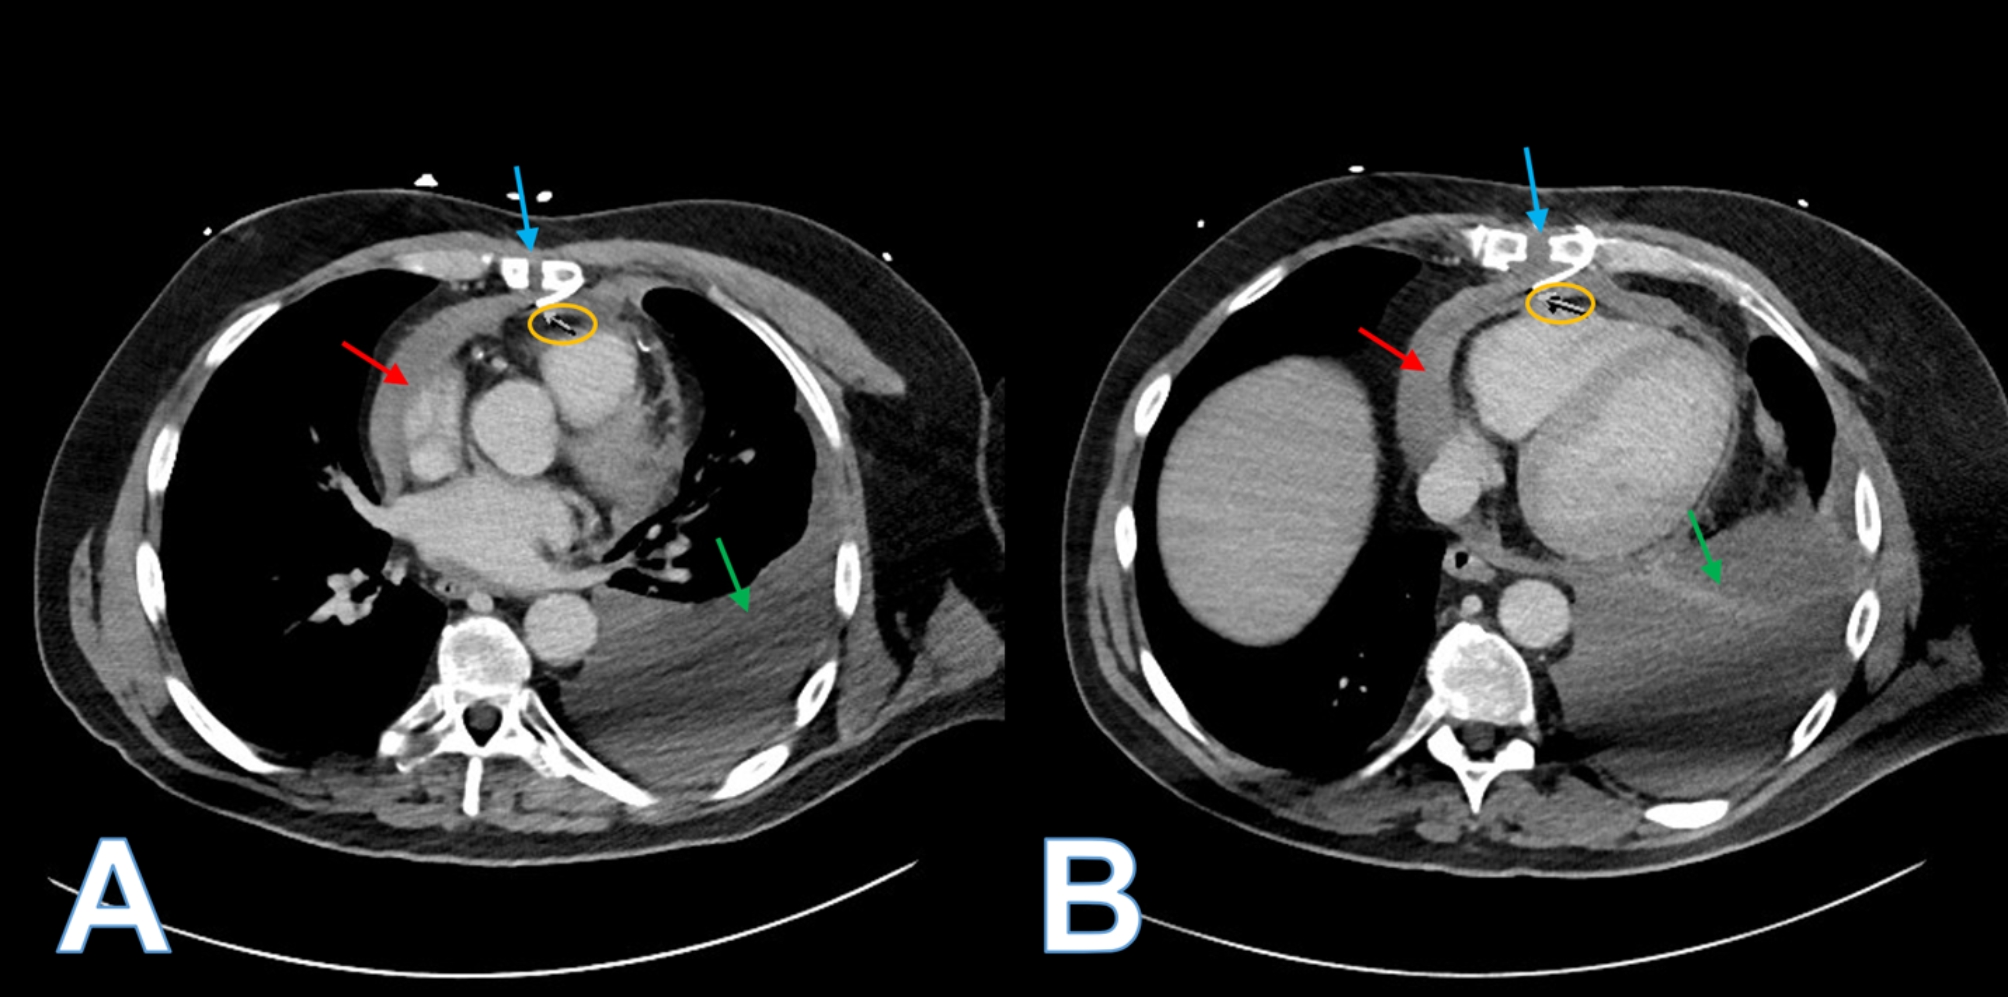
A) Computed tomography angiography at the level of the left atrium demonstrating sternal bone diastasis (blue arrow), fractured sternal wire angulating into the pericardial space (yellow circled arrow), hemopericardium (red arrow), and left pleural effusion (green arrow) B) Computed tomography angiography at the level of the diaphragm demonstrating sternal bone diastasis (blue arrow), fractured sternal wire angulating into the pericardial space (yellow circled arrow), hemopericardium (red arrow), and left pleural effusion (green arrow)
